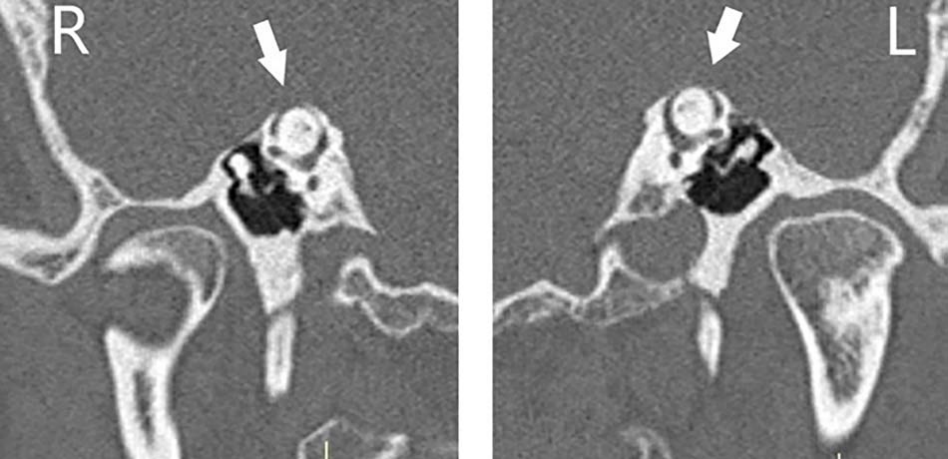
CT scan of the temporal bones of the patient, and images were reformatted in the planes of the right (R) and left (L) SSCs (Pöschl view); bilateral dehiscence (arrows) is noted.
